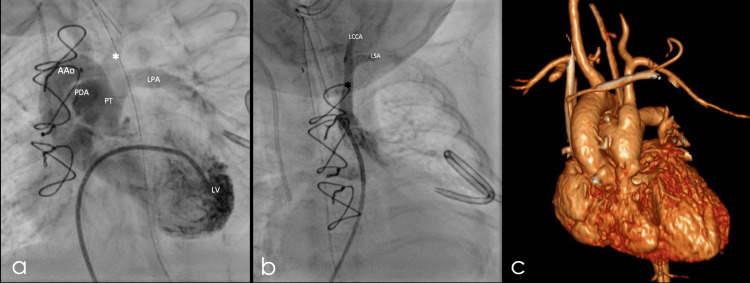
Left innominate artery. a) and b) coronal slices of cardiac catheterization confirming the CT findings of the left innominate artery (*), c) 3D reconstruction of CT angiography in the frontal plane demonstrating the same findings. LV: left ventricle, AAo: aortic arch, PDA: patent ductus arteriosus, PT: pulmonary trunk, LPA: left pulmonary artery, LCCA: left common carotid artery, LSA: left subclavian artery.
